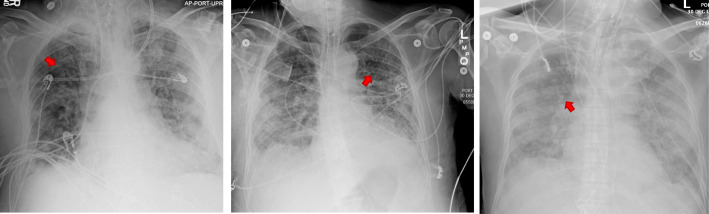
Chest X-rays of the three patients with COVID-19. Red arrows indicate the ground-glass opacities
